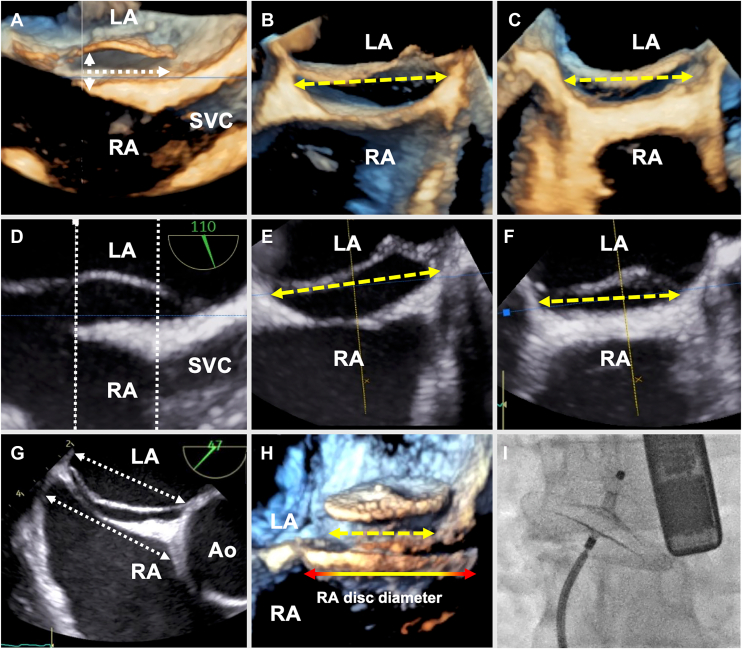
Anatomic patent foramen ovale (PFO) dimensions and tunnel width (TW) sizing by 3D transesophageal echocardiography. (A) 3D rendering of bicaval view at 110°, depicting tunnel length (horizontal dotted white arrow) and height (vertical dotted white arrow). En face 3D rendering of PFO TW_RA_ (B) and PFO TW_LA_ (C), with measurements shown with dashed yellow arrow. Bicaval 3D multiplanar image (D) at 110° of PFO tunnel, depicting transverse imaging planes (dotted white lines) used to create orthogonal multiplanar views for measurement of TW_RA_ (E) and TW_LA_ (F). (G) Midesophageal view at 47° depicting approximate atrial dimensions to ensure discs of closure device are adequately accommodated. 3D rendering (H) and fluoroscopic image (I) of PFO closure device. Ao, aorta; LA, left atrium; RA, right atrium; SVC, superior vena cava.
